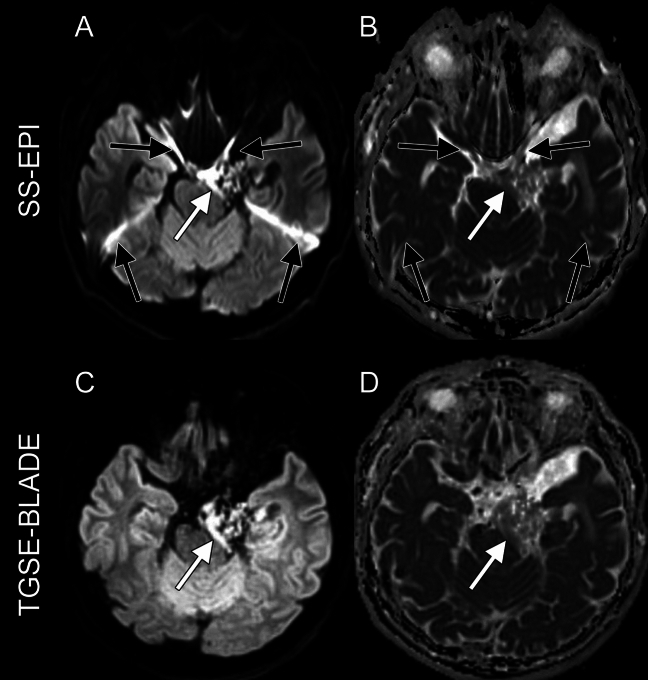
Representative images of a 62-year-old man with post-operative status of left cerebellopontine angle epidermoid cyst: b1000 and ADC from SS-EPI DWI (A, B) and from TGSE-BLADE DWI (C, D) are shown. Black arrows indicate susceptibility artifacts. Artifacts are seen near air-bone interfaces on SS-EPI DWI affecting the visualization of residual epidermoid cyst (white arrow), whereas few artifacts are seen on TGSE-BLADE DWI
